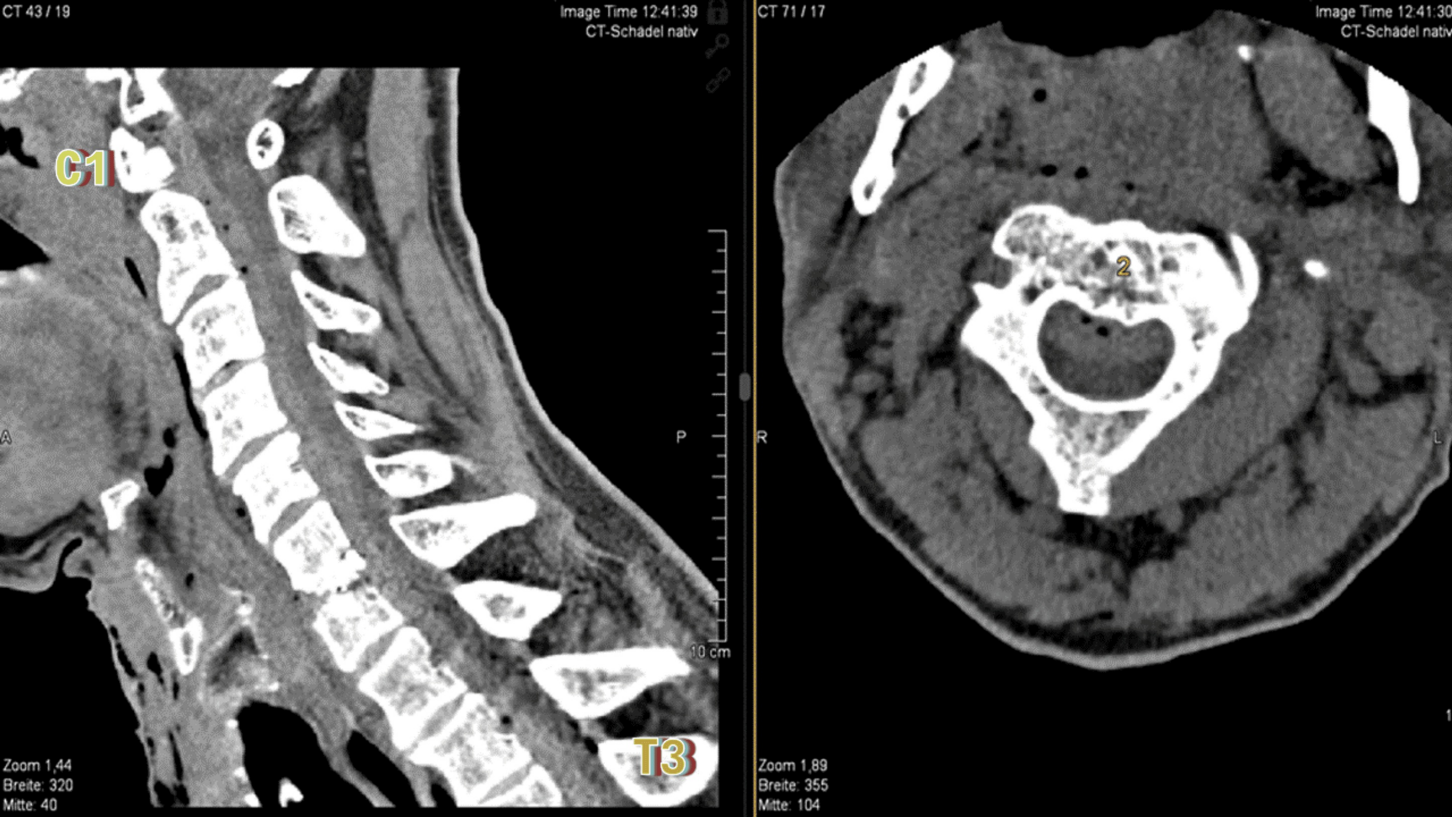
Postoperative CT imaging (sagittal and axial views) demonstrates a multilevel epidural hematoma extending from C1 to T3. CT, computed tomography
